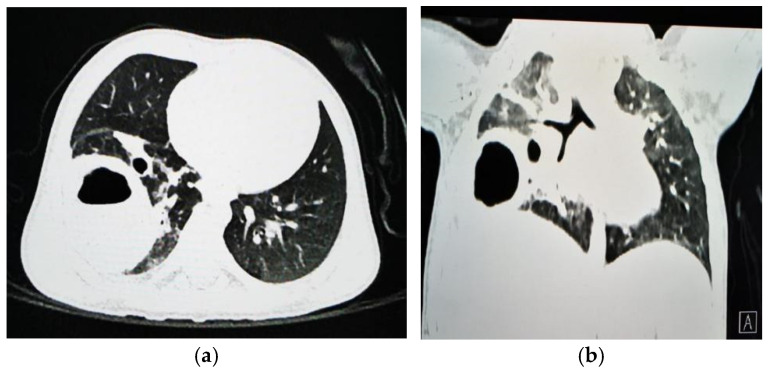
Two-year-old male child with pulmonary abscess: CT scan revealed large mass in right lower lobe, measuring approximately 53 mm × 47 mm × 50 mm, containing heterogeneous contents enclosed by thick walls. Two additional cystic lesions, measuring 13 mm and 7 mm, respectively, observed adjacent to mass without clear demarcation, indicative of multiloculated cystic lesion centered in right lower lobe; (a) transverse plane; (b) coronal plane.
